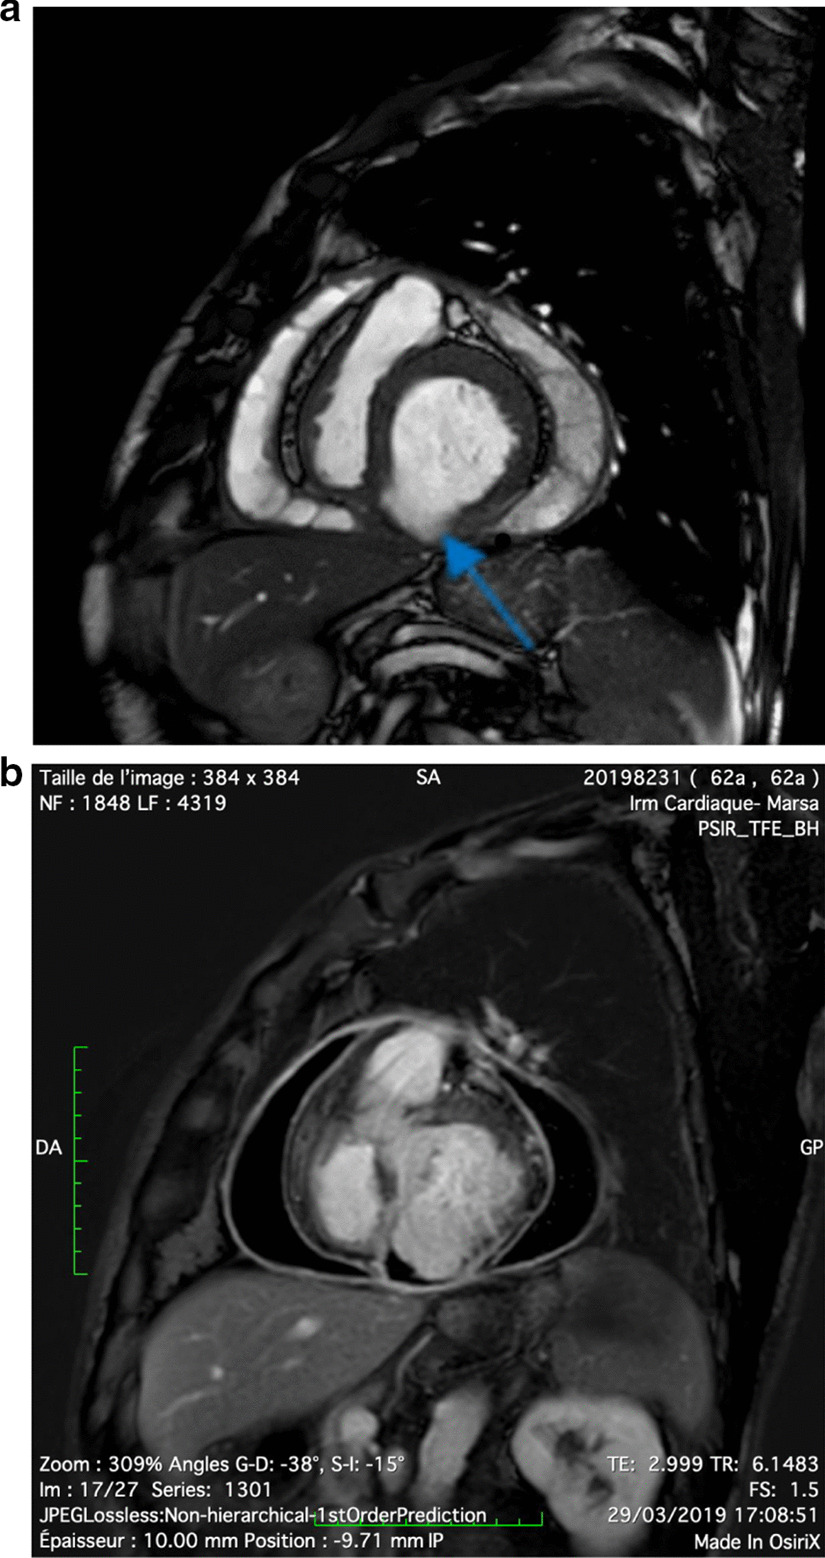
a, b Magnetic resonance imaging T2 sequence in axial section showing the false aneurysm of the inferior wall with a linear hyposignal opposite the endocardium corresponding to the thrombus (shown by the blue arrow) with huge hemopericardium
